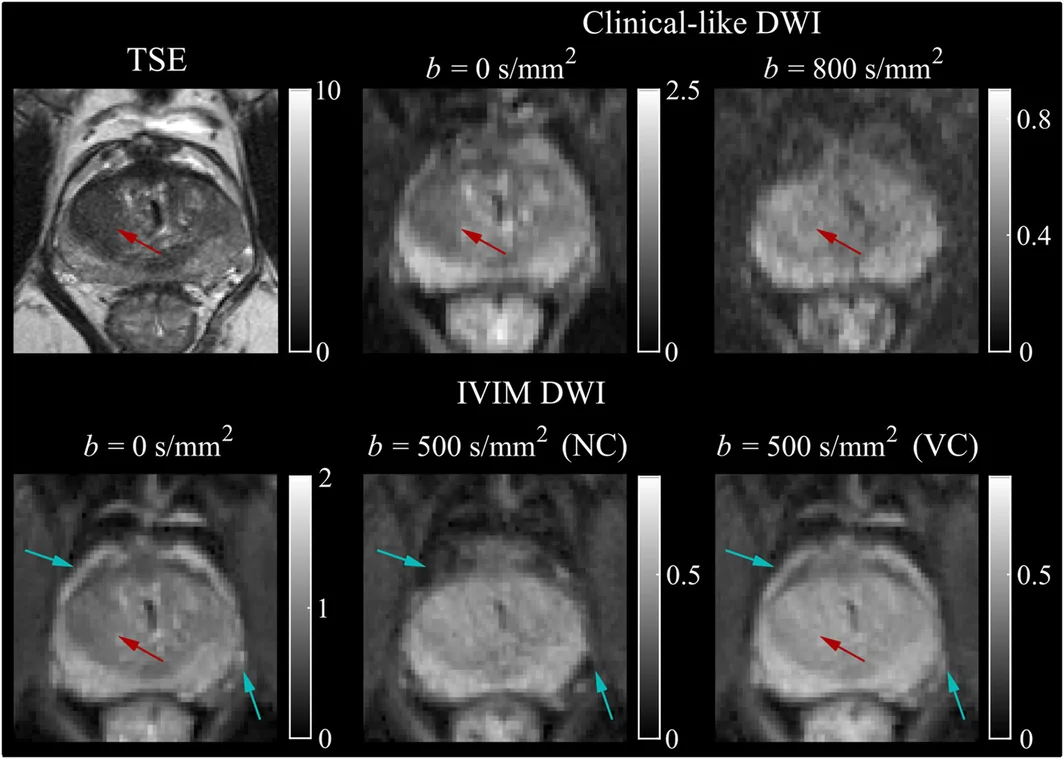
Examples of a standard ‘clinical‐like’ diffusion‐weighted imaging (DWI) protocol (top row) and the proposed IVIM DWI protocol (bottom row; NC—non–velocity‐compensated, VC—velocity‐compensated) from patient ID = 2. All images were averaged over repetitions (b‐values and directions). The geometric consistency of fine anatomical features was well preserved in IVIM data, while ‘clinical‐like’ data had relatively low spatial resolution and more prominent artifacts due to eddy currents and local field inhomogeneity. The red arrow points to a cancerous lesion in the central zone, and blue arrows point to the venous plexus (anterior) and neurovascular bundle (posterior) around the prostate gland, which were most visible when using velocity‐compensated encoding.
